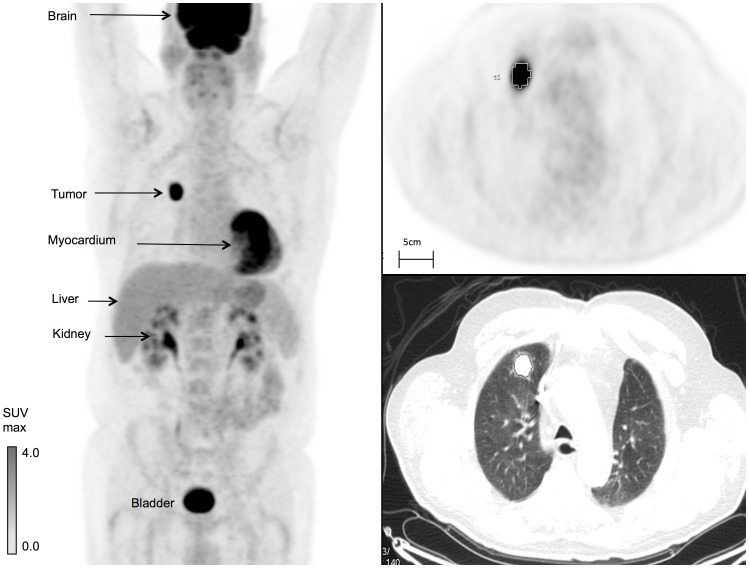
Non-small Cell Lung Cancer FDG PET-CT Imaging Features. A three dimensional, maximum intensity projection, whole body ^18^F-FDG PET-CT (left). Physiologic uptake is seen in the brain, heart and liver with excretion through the renal pelvis and bladder. This tumor showed an intense FDG uptake with SUV_max_ of 19, SUV_mean_ of 9.6, and TLG of 65.6 using a 50% SUV_max_ threshold (upper right). On CT, the lesion volume was estimated at 6.0 cm^3^ with a maximum diameter of 22 mm (lower right).
